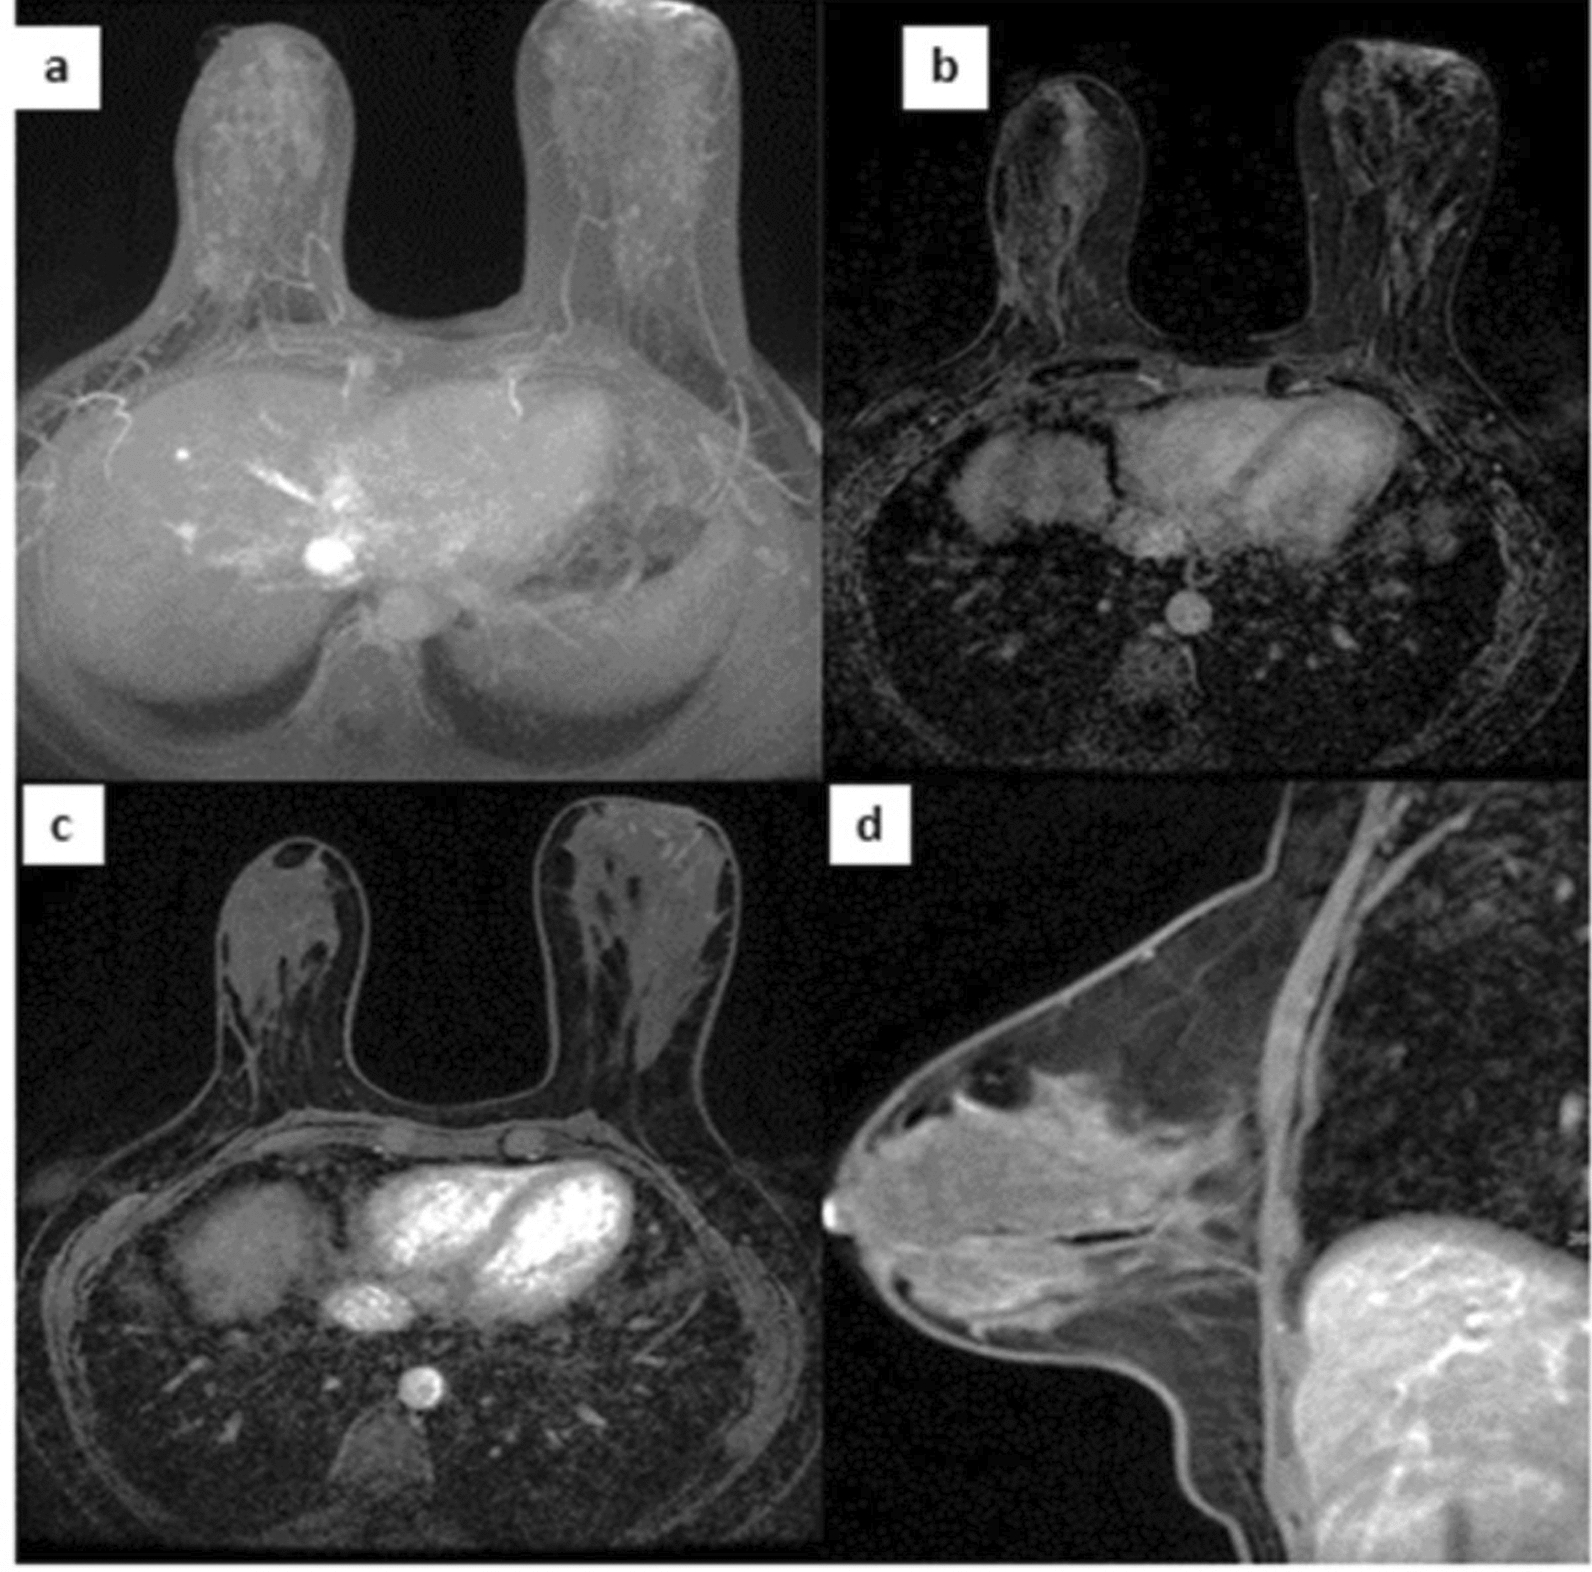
Magnetic resonance imaging performed 9 months after start of TB therapy: a MIP on axial plane, b post contrast subtracted T1-weighted image with fat saturation and c and no-subtracted on axial plane and d sagittal plan show a significative reduction in size of the enhancing tissue, with multiple and confluent foci of contrast enhancement. The skin thickenning has also disappeared. *MIP* Maximum intensity projection
